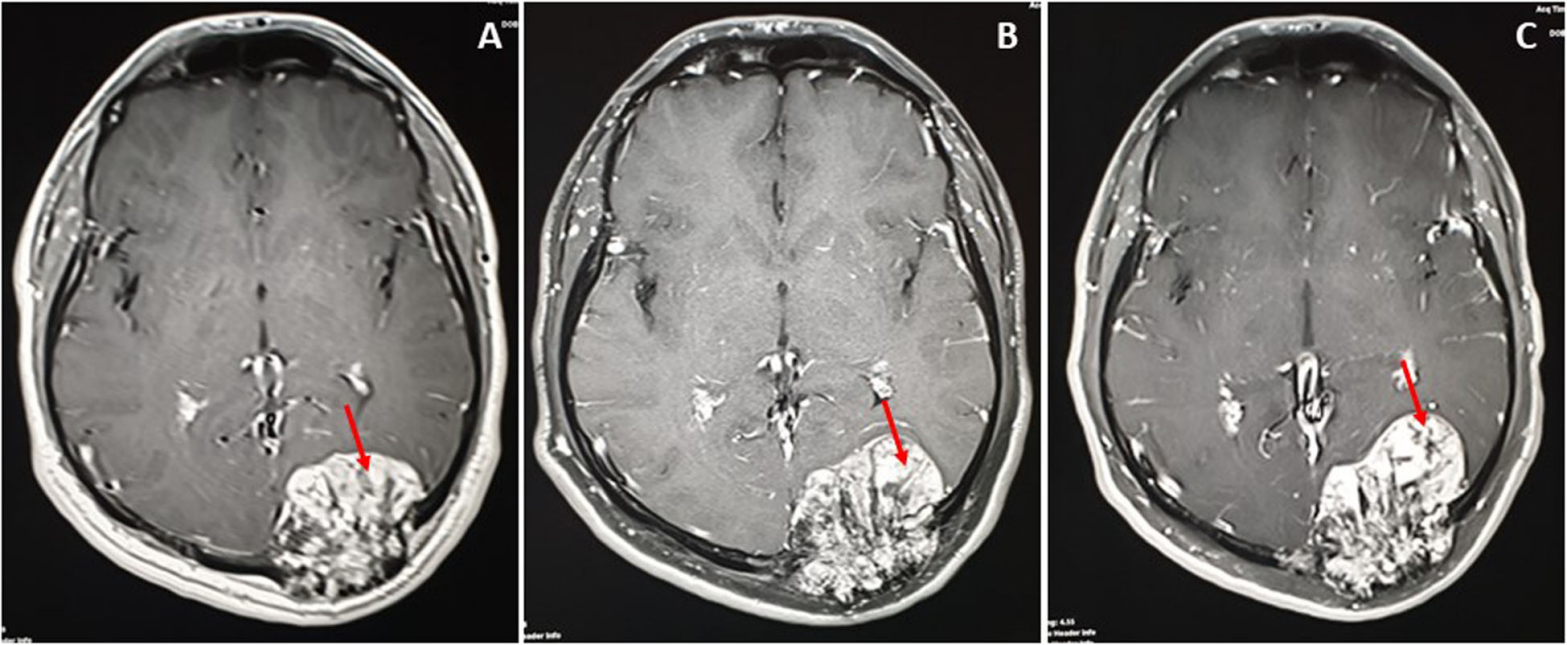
Serial axial MRI (post contrast T1) done on February 2017 (a); January 2018 (b) and April 2019 (c), showing progressive enlargement of the soft tissue component and increased mass effect
